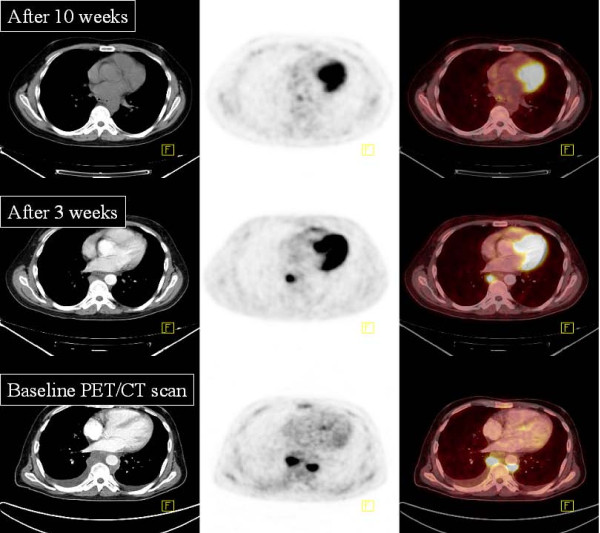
18F-FDG- PET/CT findings. Baseline: At presentation high metabolic activity in mediastinal mass. After 3 weeks: Both structural and metabolic regression. After 10 weeks: Complete structural and metabolic remission.
